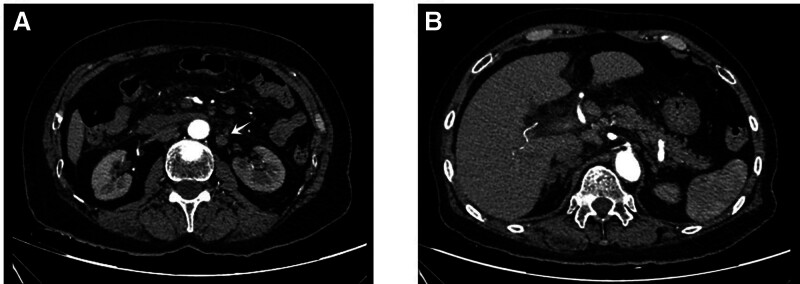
Enhanced-contrast computed tomography of the patient with pulmonary tumor microangiopathy as the disease aggravated. (A) Multiple lymph nodes were enlarged in upper abdomen and retroperitoneum. (B) The peripancreatic space was blurred.
